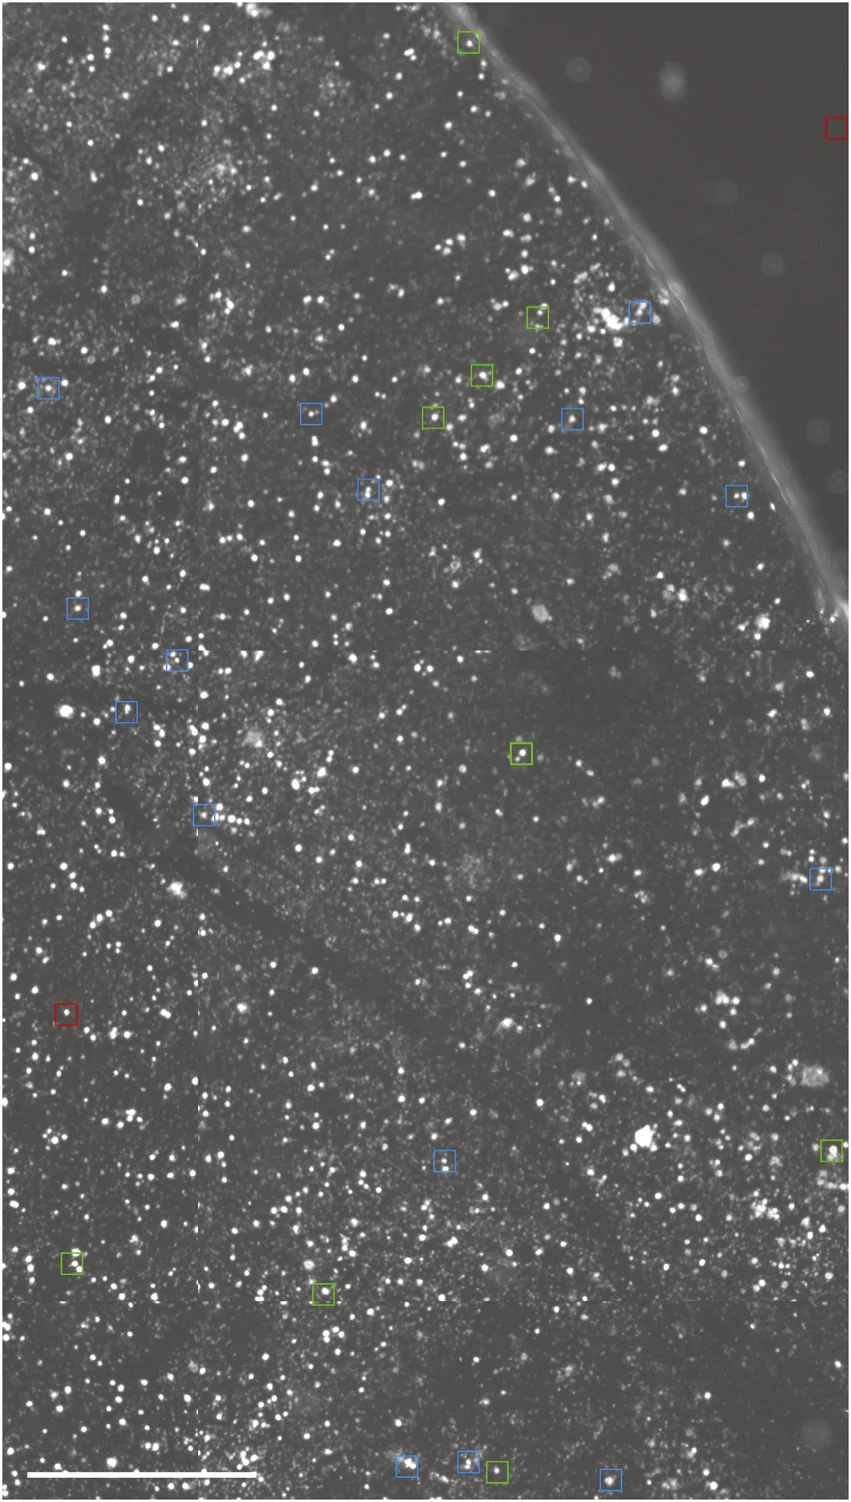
PSMA labeling in an 8 mm coverslip of peripheral blood. Metastatic patient CTCs are detected as PSMA+/DAPI+/CD45- blobs. Magnification 10×. Scale bar equals 450 nm. The figure shows the same coverslip and detections as in Figure 6, but only a small portion of the upper-right corner of the PSMA channel is shown with overlaid detections. Green squares mark the CTCs (TP). Blue squares mark the leukocytes (TN). Red squares mark the FP. Note the FP in the upper-right corner of the image, resulting from the border effect between adjacent tiles. A larger size version of this image can be viewed at: https://github.com/amatov/SegmentationBiomarkerCTC/blob/main/Coverslip/p001_1crop.jpg.
